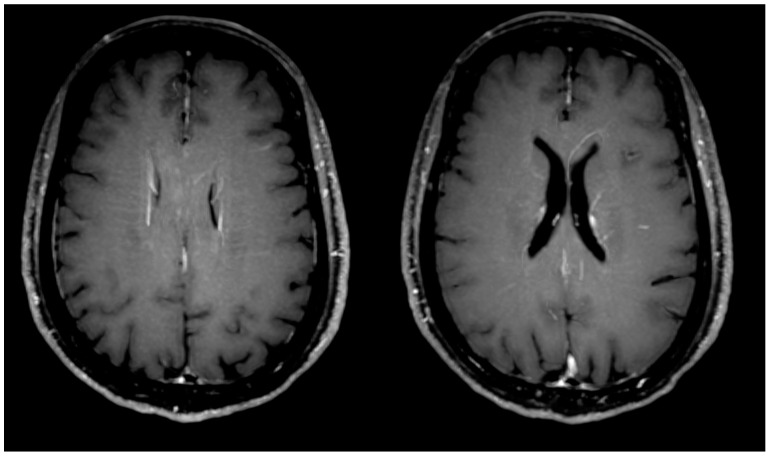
Axial MIP reconstruction of the post-contrast 3D T1-weighted images at the level of lateral ventricles shows bilateral perivascular enhancement along engorged deep medullary veins.
